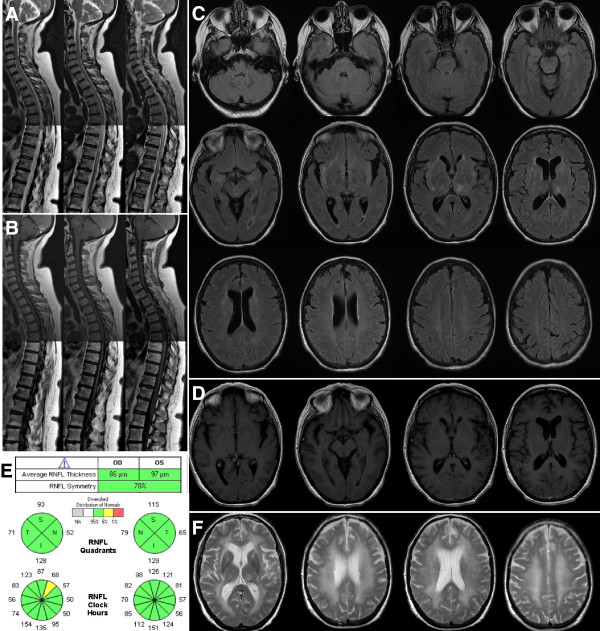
Spinal cord, brain and optical imaging follow-up of the patient. June 2012: Spinal cord magnetic resonance imaging (MRI) showing extensive hyper intense T2 lesion between C5 and T10 (A) with gadolinium enhancement on T1 sequence (B). Brain MRI showing multiple hyperintense T2 lesions on insular regions, medial temporal lobes and thalamus (C), but also gadolinium enhancement of ventricles and meninges (D). Peripapillary optical coherence tomography showing asymmetric retinal nerve fiber layer (E). July 2012: Control brain MRI showing extension of hyperT2 lesion load (F).
